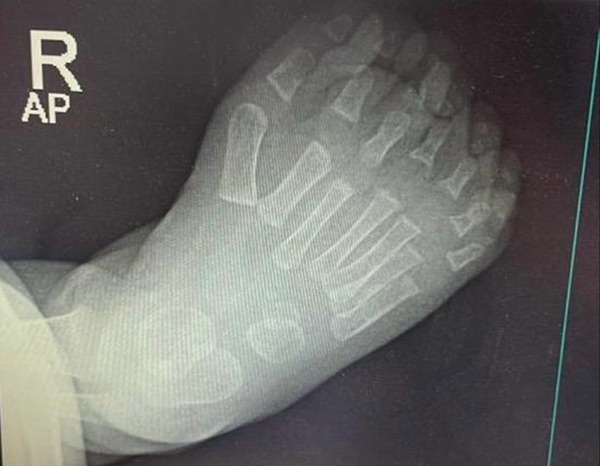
An anteroposterior radiograph of the right foot showing complete duplication of the medial and lateral rays with independent metatarsals and phalanges. No central ray duplication or tarsal abnormalities are identified.
